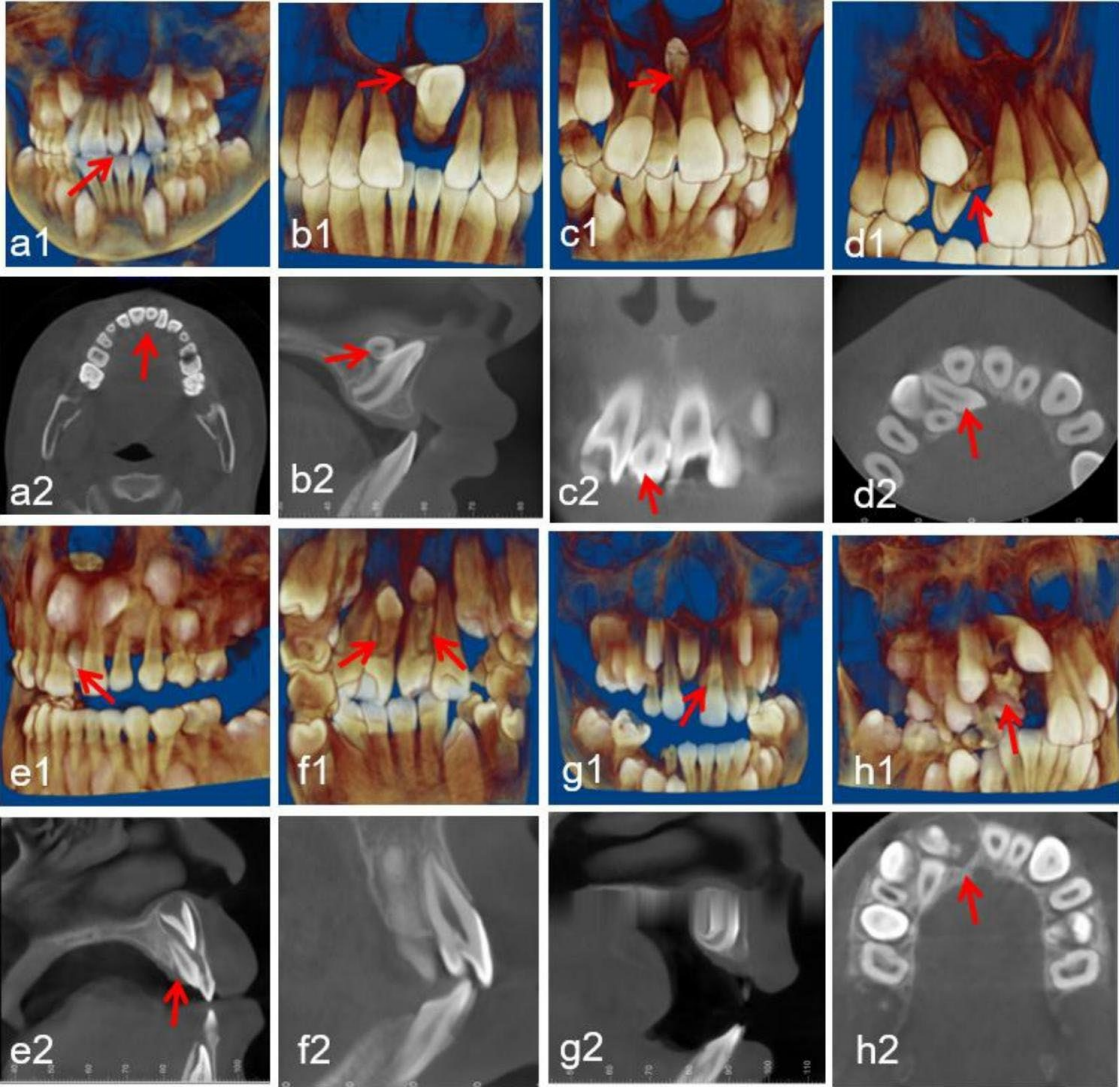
Representative CBCT images exhibiting diverse ST-associated complications of adjacent teeth. (a1-a2) rotation; (b1-b2) impacted eruption and delayed development; (c1-c2) median diastema; (d1-d2) displacement; (e1-e2) root resorption; (f1-f2) enamel invagination; (g1-g2) curved root; (h1-h2) cyst formation. The red arrows point to the ST in the CBCT images
